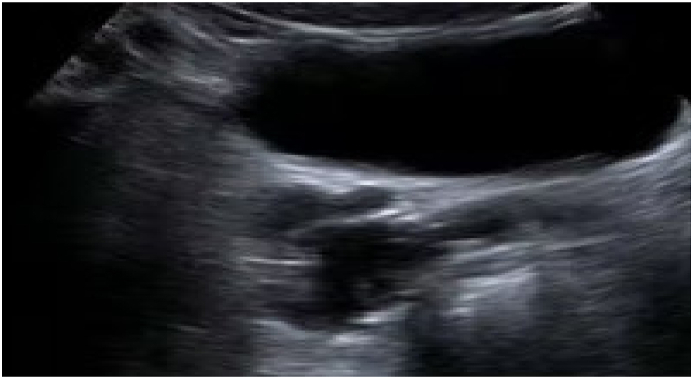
Ultrasound showing a cystic mass of the right seminal vesicle.
